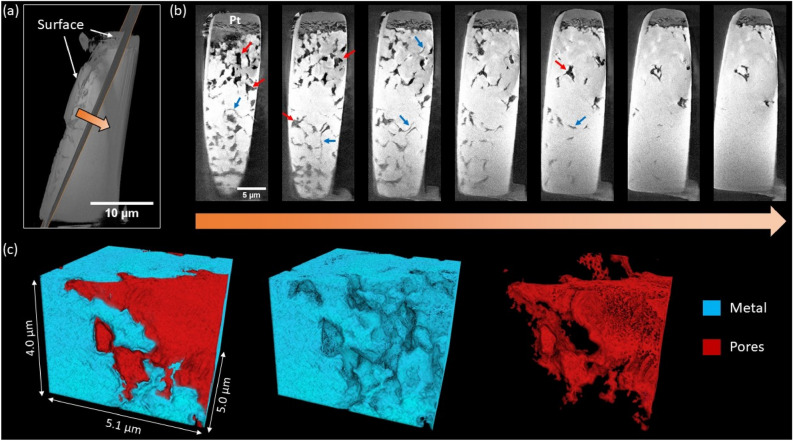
Synchrotron X-ray nano-tomography on Ni–20Cr corrosion in KCl–NaCl in air for 16 h: (a) Diagonal slice of interest used for depth profiling. (b) Pseudo-2D cross sections along depth direction, spaced approximately 0.4 μm apart. Some larger pores and intergranular cracks are indicated using red and blue arrows, respectively. (c) 3D volume rendering of near-surface region displaying pore formation.
